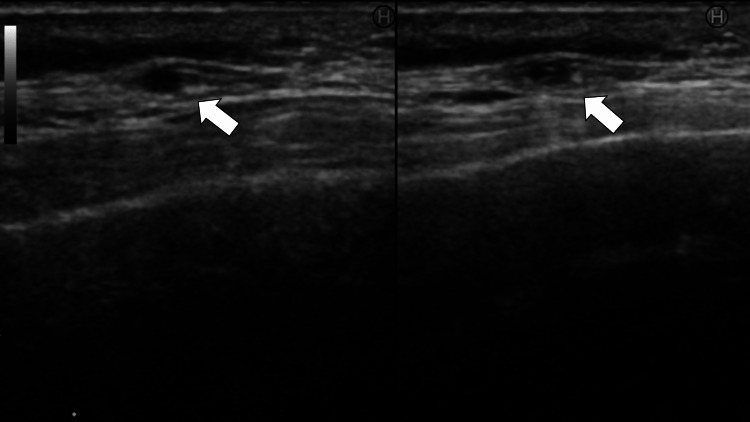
Ultrasound examination on temporal artery revealing halo signs in the compression on the artery (white arrows)
